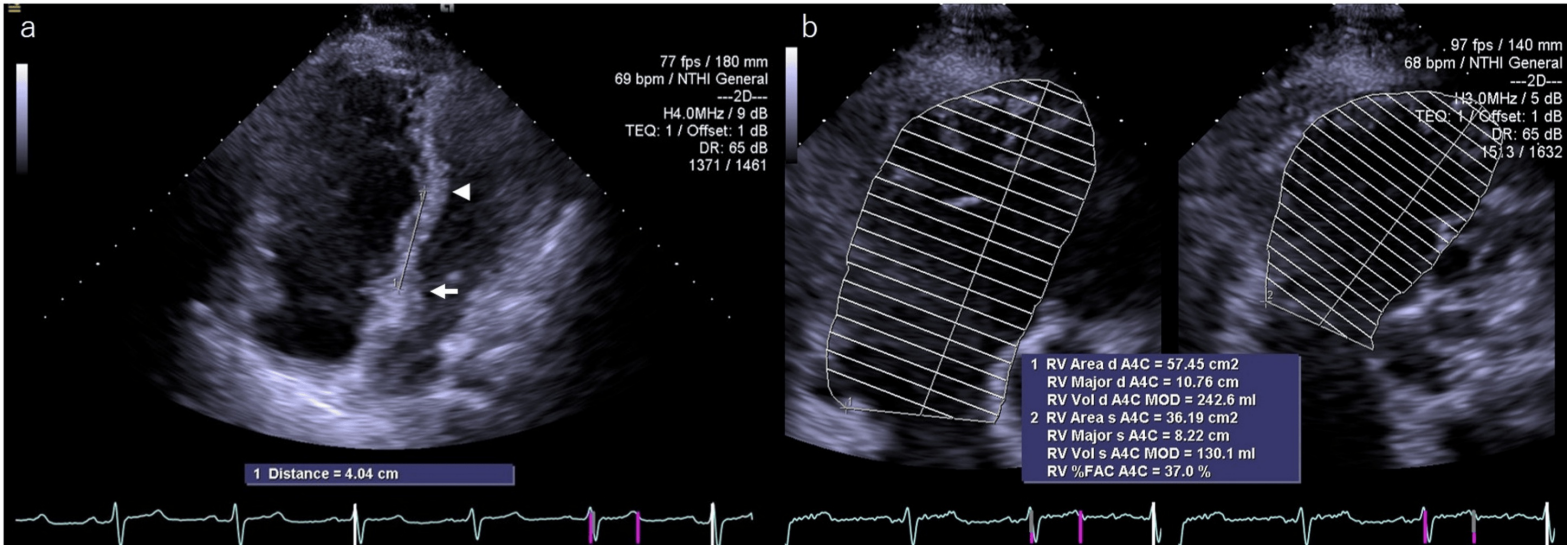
Preoperative transthoracic echocardiography in Case 2 (a) The septal leaflet of the tricuspid valve was displaced approximately 40 mm apically from the anterior mitral leaflet. The arrow marks the position of the anterior mitral leaflet, and the arrowhead indicates the location of the tricuspid septal leaflet. (b) Preoperative assessment of right ventricular function. RV Area d; Right ventricular end-diastolic area; A4C: apical four-chamber view; RV Major d: major axis diameter in diastole; RV Vol d: right ventricular end-diastolic volume; MOD: modified Simpson’s method; RV Area s: right ventricular end-systolic area; RV Major s: right ventricular major axis diameter in systole; RV Vol s: right ventricular end-systolic volume; RV %FAC: right ventricular % fractional area change
